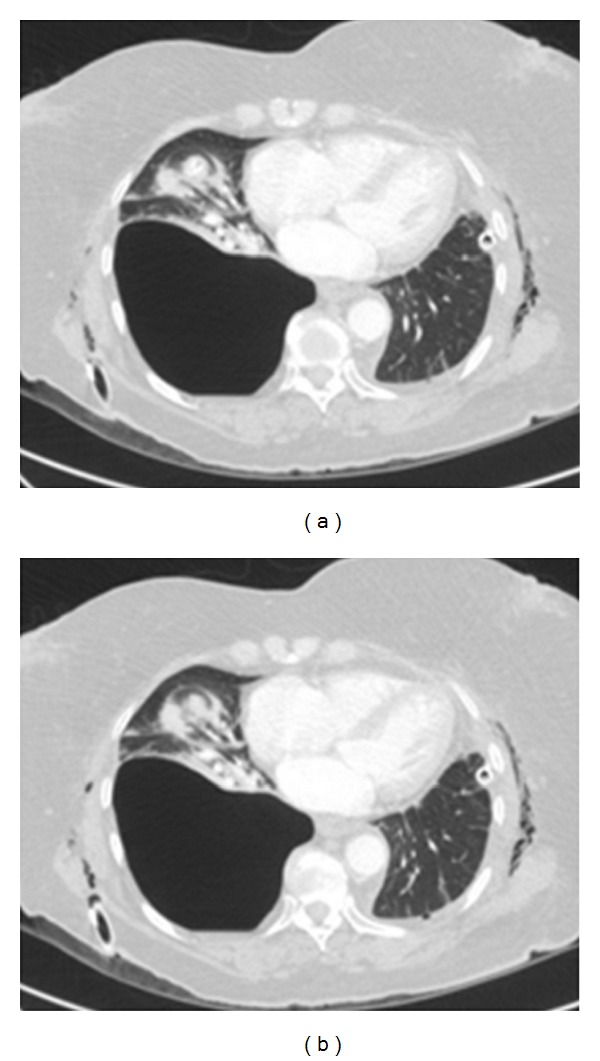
Axial CT scans on the same patient as in Figure 29 showing a large bulla in the right lower lobe associated with passive atelectasis in the right middle lobe. Note the surgical emphysema.
